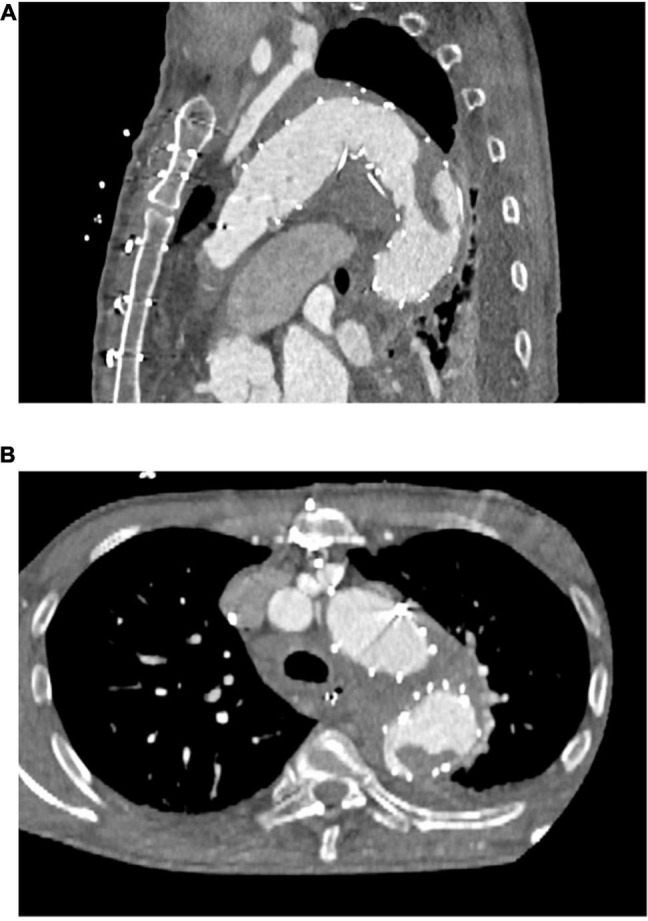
Representative computed tomography angiographic images of a newly detected postoperative thrombus formation within the frozen elephant trunk stent-graft. (A) Sagittal plane (B) axial plane.
